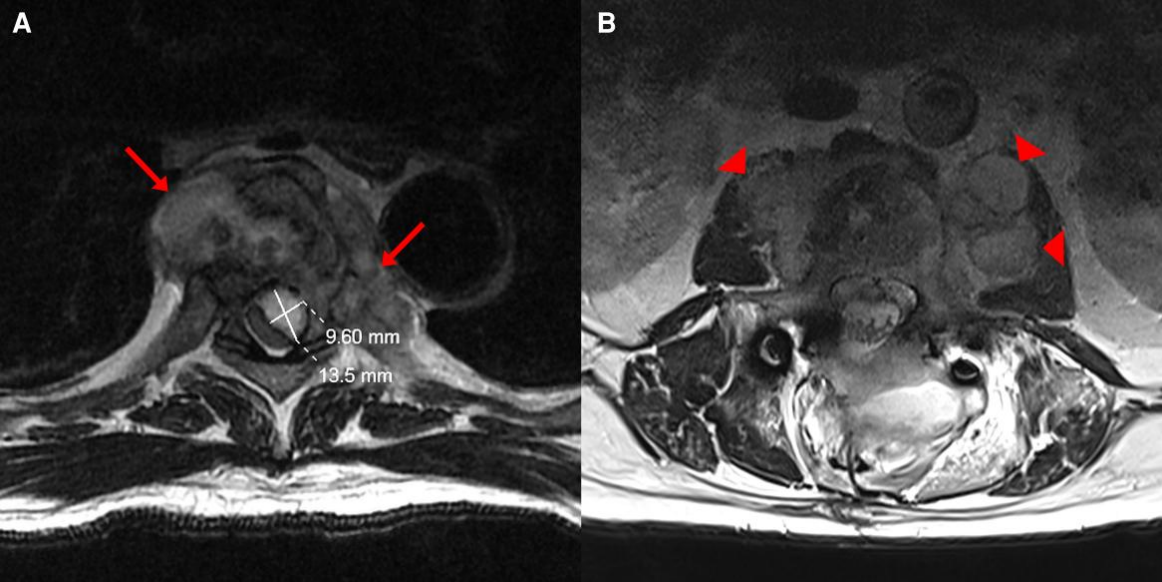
At the time of diagnosis, T2-weighted magnetic resonance imaging of vertebra T6 (*A*) and the evacuated vertebra L2 (*B*) in axial views. The arrows highlight paravertebral abscesses in the thorax. The cross mark outlines the dimensions of the thoracic epidural abscess as 9.60 × 13.5 mm. The arrowheads highlight the paravertebral abscesses at the psoas muscles.
